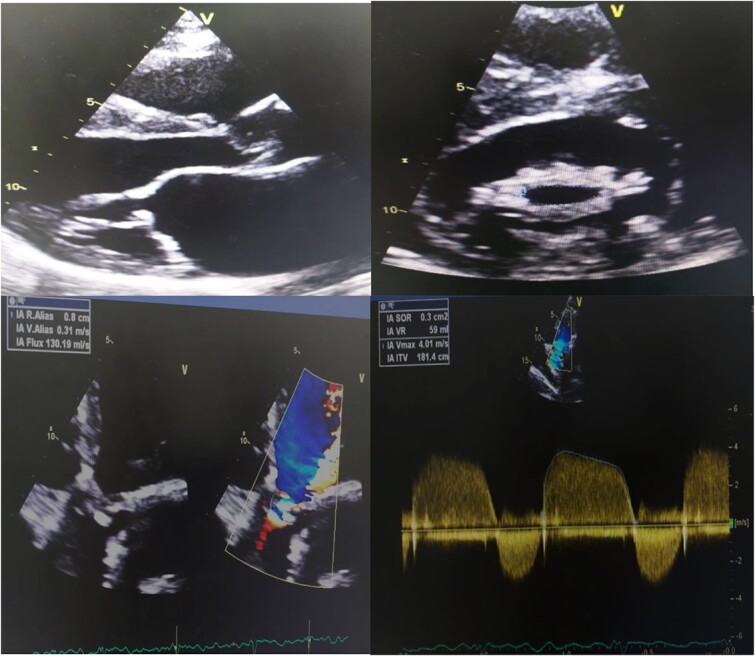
A transthoracic echocardiography showed severe mitral stenosis with severe aortic regurgitation and biatrial enlargement. It also reveals segmental ventricular wall motion abnormalities: akinesis of the apex and basal and medial anterolateral wall with preserved fraction ejection at 50%.
